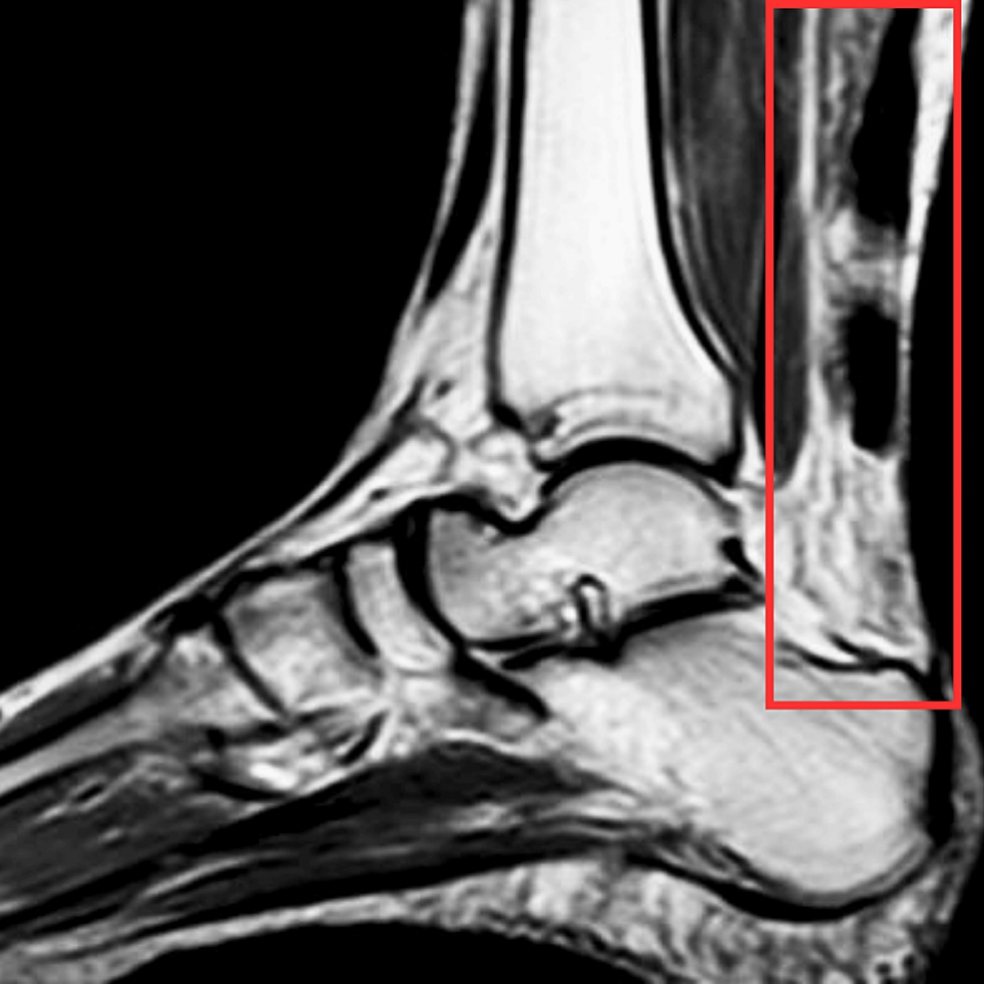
MRI of the ankle joint The rectangle shows a ruptured AT. AT, Achilles tendon
